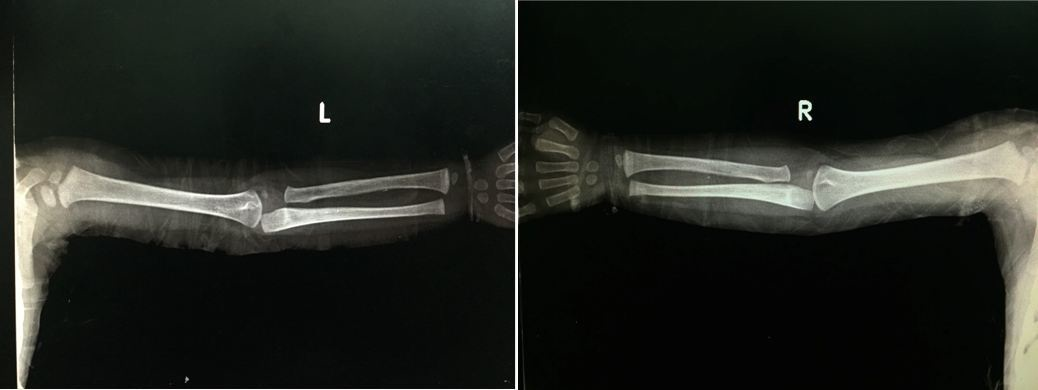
Anteroposterior (AP) view of left and right upper limb Follow-up radiographs of the same child after 2.5 years show complete resolution of periosteal new bone formation in previously involved left radius and right ulna.
